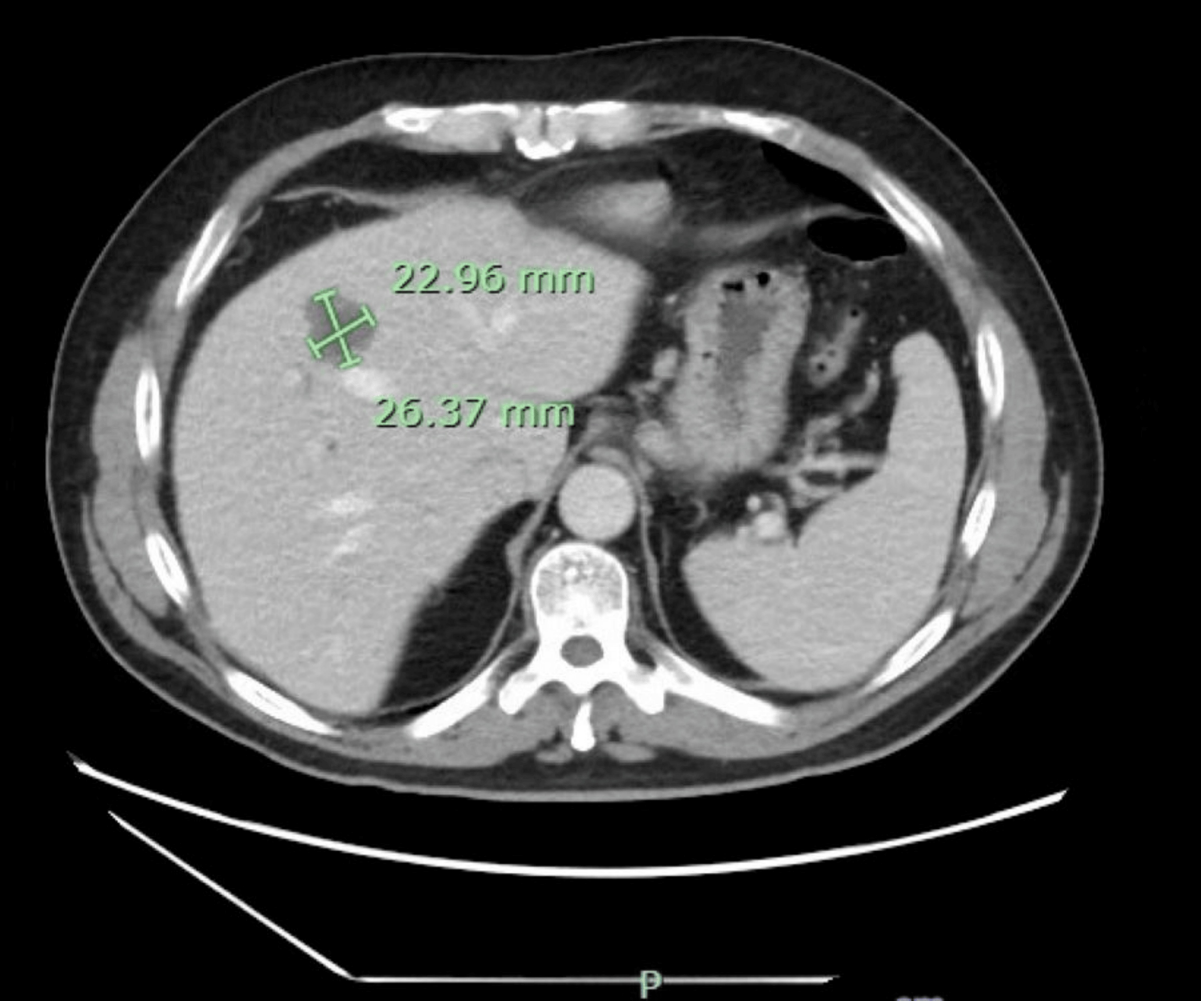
Contrast-enhanced CT of the abdomen at a different slice demonstrating a second hepatic lesion concerning for possible malignancy, with a subtle nodular liver contour suggestive of early cirrhosis.
